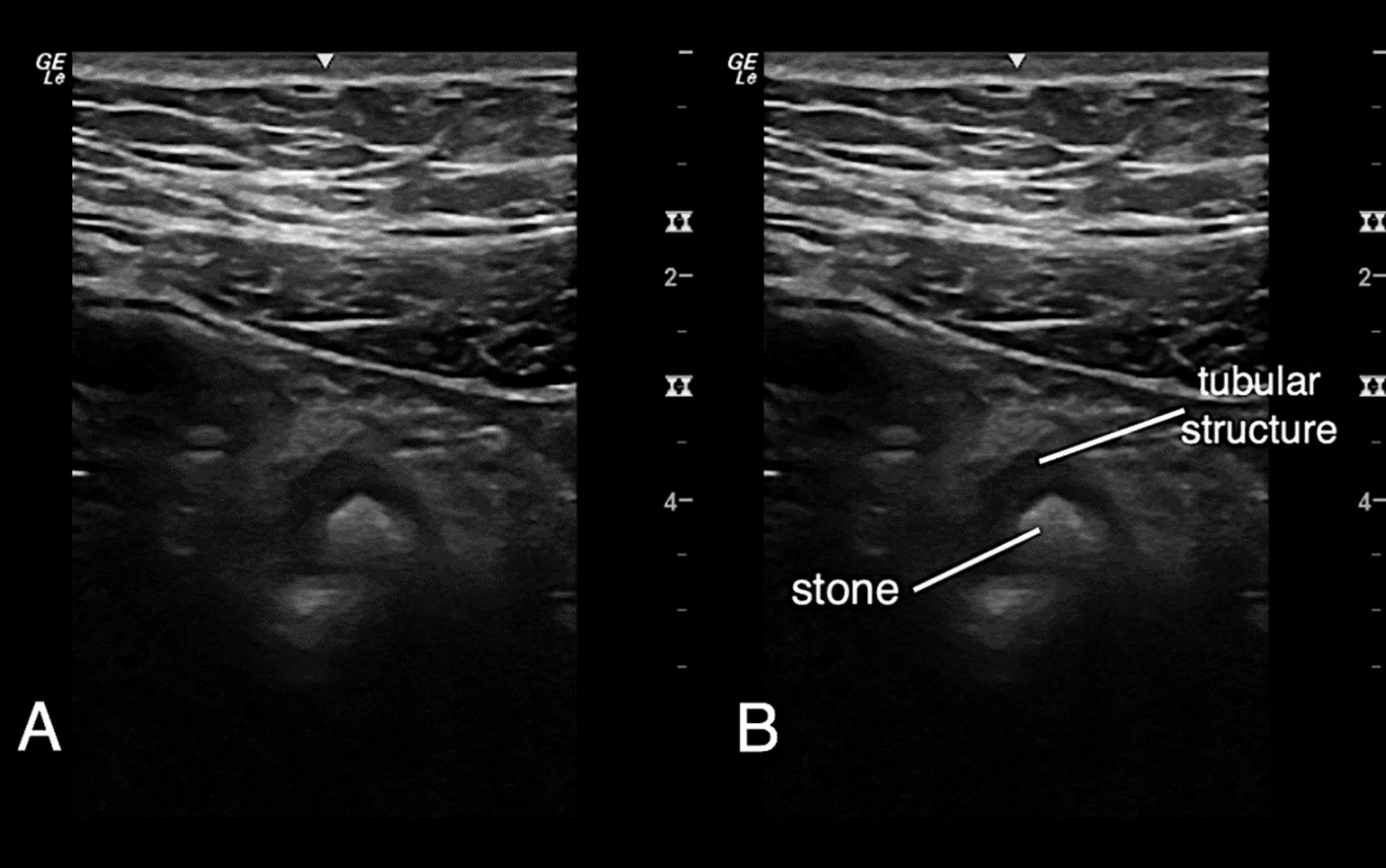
Ultrasound right lower quadrant, short axis, high frequency linear probe A hyperechoic circular object is noted within a hypoechoic tubular structure. The hyperechoic object is a ureteral stone noted within a dilated ureter.
